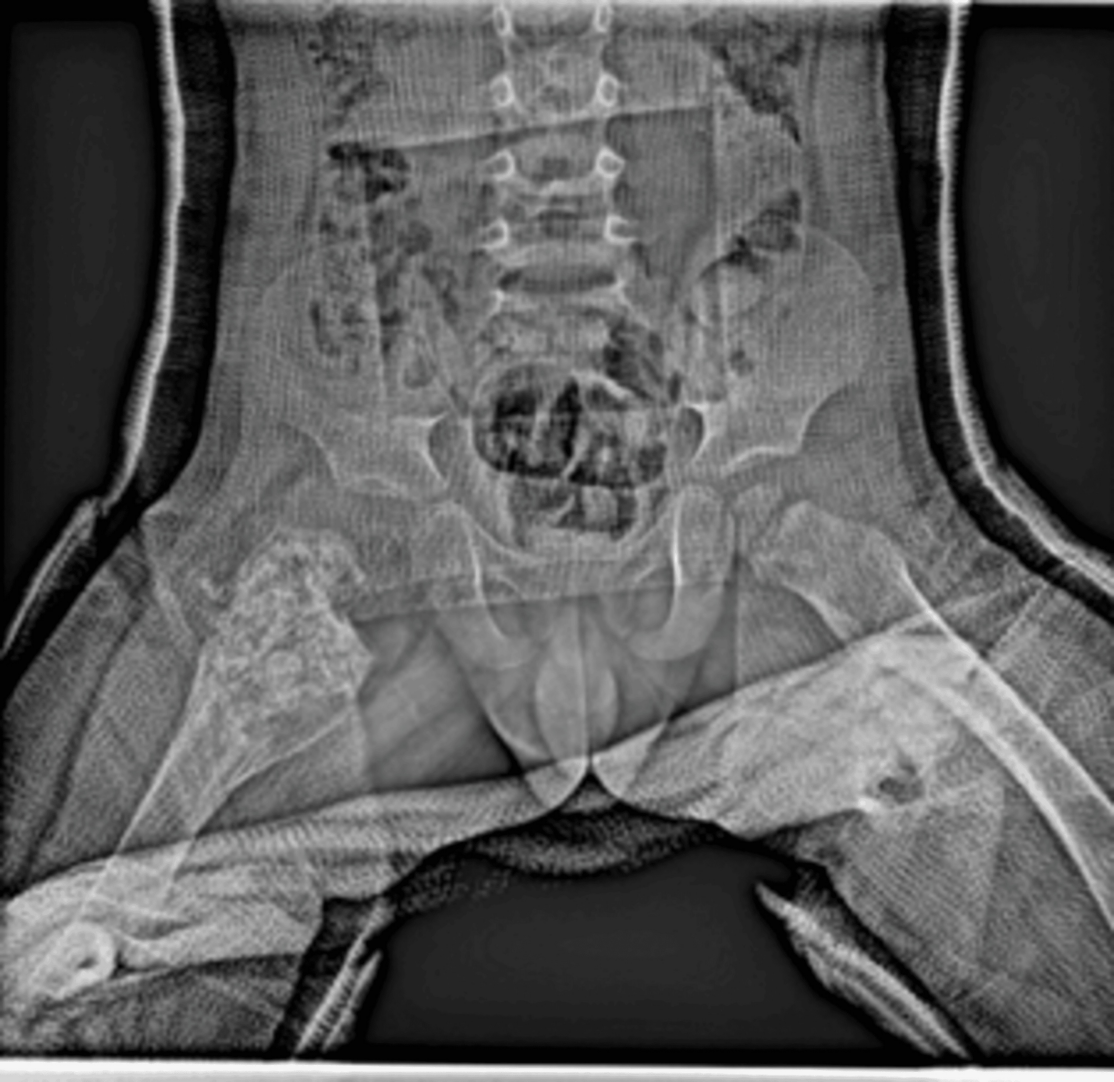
Postop sixth-week control radiograph
